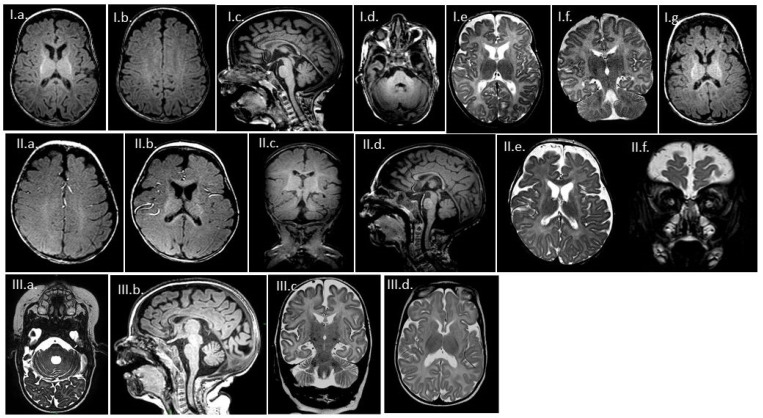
Brain MRI images of Patients 1, 2, and 4. Patient 1: T1 weighted (I.a–d) and T2 weighted (I.e,f) images at the age of 5 months and 8 months (I.g) on T1 sequence; Patient 2: T1 (II.a–d) and T2 (II.e,f) weighted images at 8 months of age; Patient 4: T2 weighted axial image at 4 months of age (III.a), T1 sagittal image (III.b), T2 coronal and axial planes (III.c,d).
